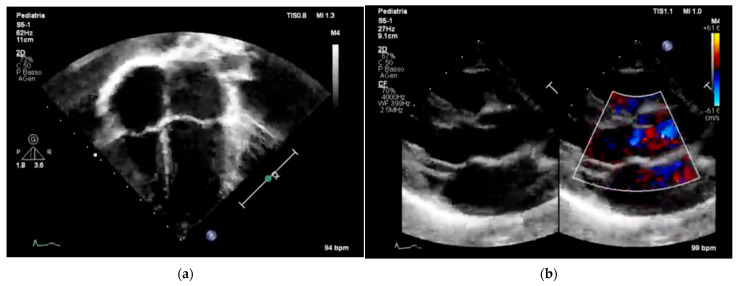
Two representative examples of echocardiography in pediatric subjects: (a) four-chamber pediatric view with apex down in 6-year-old children; (b) parasternal long axis view of a 6-year-old child; black and white on the left and color Doppler flow on the right.
